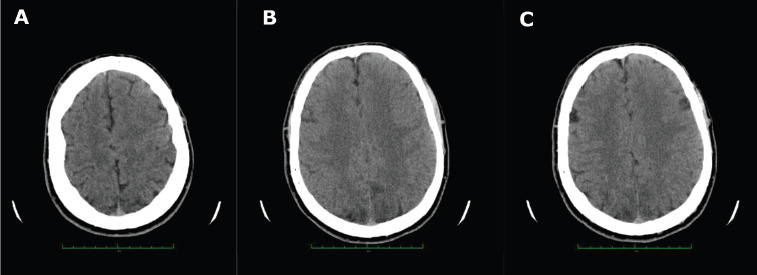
Head computed tomography findings suggestive of PRES: bilateral cortical-subcortical hypodensities in parietal and occipital lobes (A, B, C)
